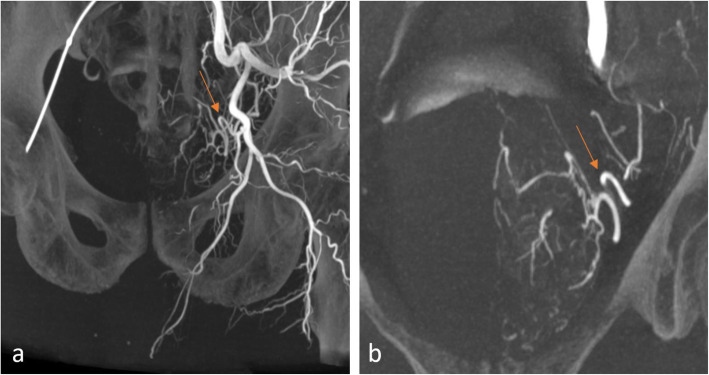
Proximal CBCT. Five seconds CBCT acquisition, injection from left internal iliac artery (Discovery IGS 740, GE Healthcare, Chicago, IL). Maximum Intensity Projection (MIP) coronal views showing the left pelvic arterial vascular anatomy (a) and the left prostatic artery (arrow) and prostate gland blush (b)
